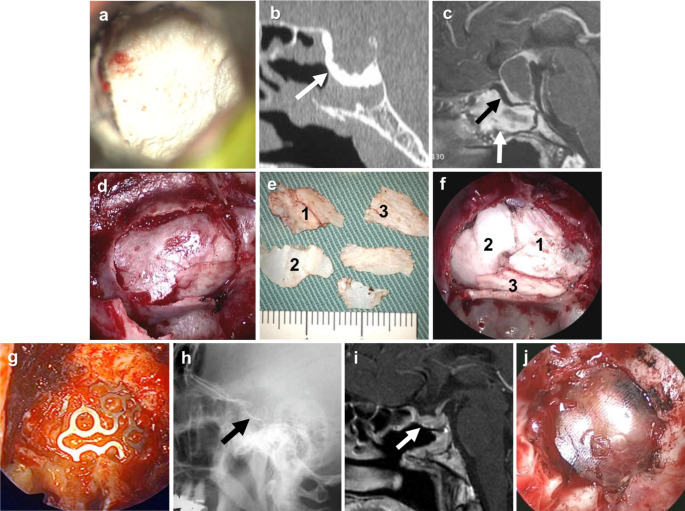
(a) Intraoperative view showing rigid sellar floor reconstruction using calcium phosphate cement (CPC) paste. (b) Sagittal bone-window CT scan demonstrating CPC paste reconstruction of the sellar floor (white arrow). (c) Contrast-enhanced sagittal T1-weighted MRI showing CPC paste reconstruction (black arrow) and inflammatory changes within the sphenoid sinus (white arrow). (d) Intraoperative view of sellar floor reconstruction using a single nasal septal bone fragment. Gelatin sponge was placed in the epidural space, and the bone fragment was inserted extradurally beneath the bony edge of the sellar window. (e) Harvested nasal septal bone fragments prepared for reconstruction. (f) Sellar floor reconstruction using three bone fragments, each corresponding to the numbered fragments shown in panel e. (g) Intraoperative view of sellar floor reconstruction using a titanium mesh plate. (h) Lateral radiograph demonstrating the titanium mesh plate (black arrow). (i) Contrast-enhanced sagittal T1-weighted MRI demonstrating the titanium mesh plate (white arrow). (j) Intraoperative view of sellar floor reconstruction using a resorbable fixation plate (Lactosorb^®^)
